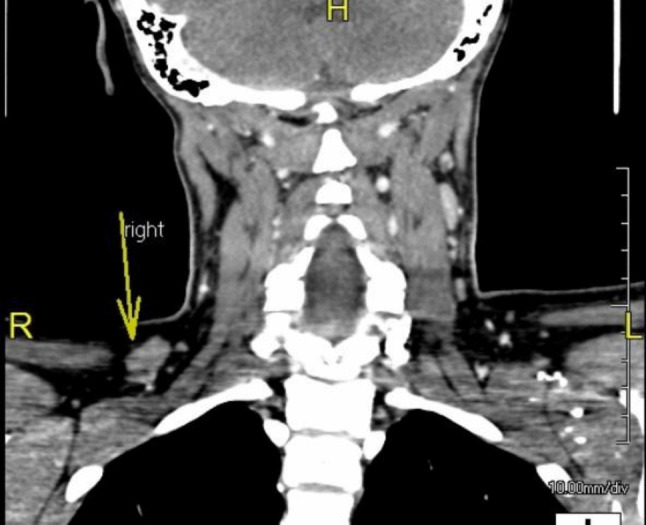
Radiographical finding of right enlarged cervical lymph node during 2025 hospitalization
